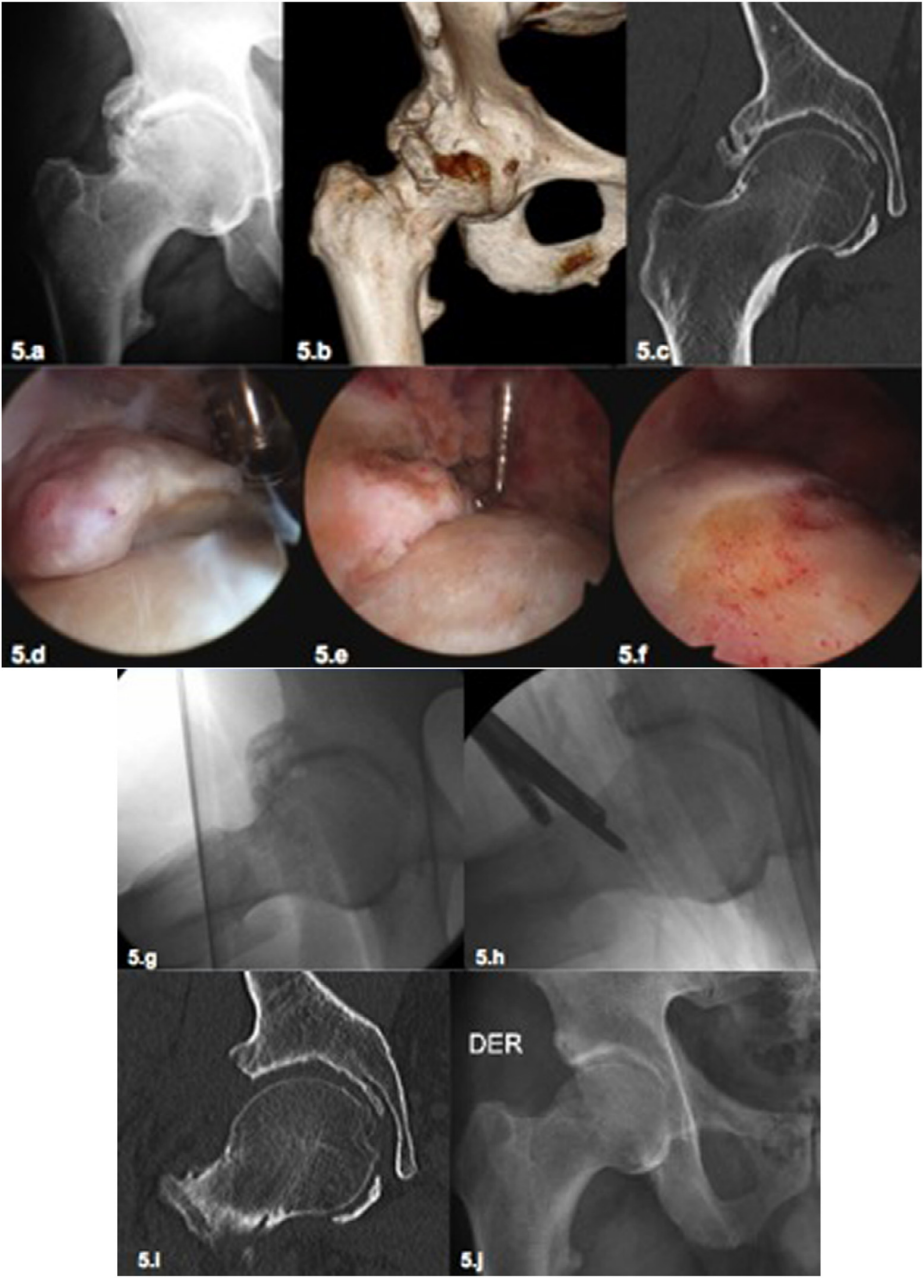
(5a–5f) Male patient, 65 years with an advanced osteoarthritis of the hip with a significant ROM (5a). The patient and his wife (orthopedic surgeon) refused the proposed treatment with a total joint replacement insisting to perform a hip arthroscopy. The complemental imaging studies with CAT Scan demonstrate the extensive osteophytes (5b) with conservation of the joint space in the load-bearing area (5c). After a long discussion with the patient without creating false expectations, a hip arthroscopy was performed. The osteophytes in the peripheral compartment were resected and the acetabular labrum was debrided (5d, 5e). The acetabular rim was resected as well as the existing cam deformity (5f). (5g–5j) The same patient from Figures 5a–5f. The intraoperative radioscopy demonstrated the satisfactory resection of the osteophytes and the bone deformity (5g, 5h). The postoperative CAT scan (51) and the postoperative X-ray (5j) demonstrated also a satisfactory result. Five years after this surgery the patient has still an excellent clinical outcome (HHS 92) without progression of osteoarthritis. This case demonstrates that we still do not know where exactly are the limits for hip arthroscopy in cases with osteoarthritis. It demonstrated also that in advanced pincer cases with arthritis due to the resection of the deformity, good results can be achieved (5i, 5j). Independent of this satisfactory clinical result, in cases like these a total joint replacement (TJR) is the most effective and predictable option of treatment but it was refused by the patient.
